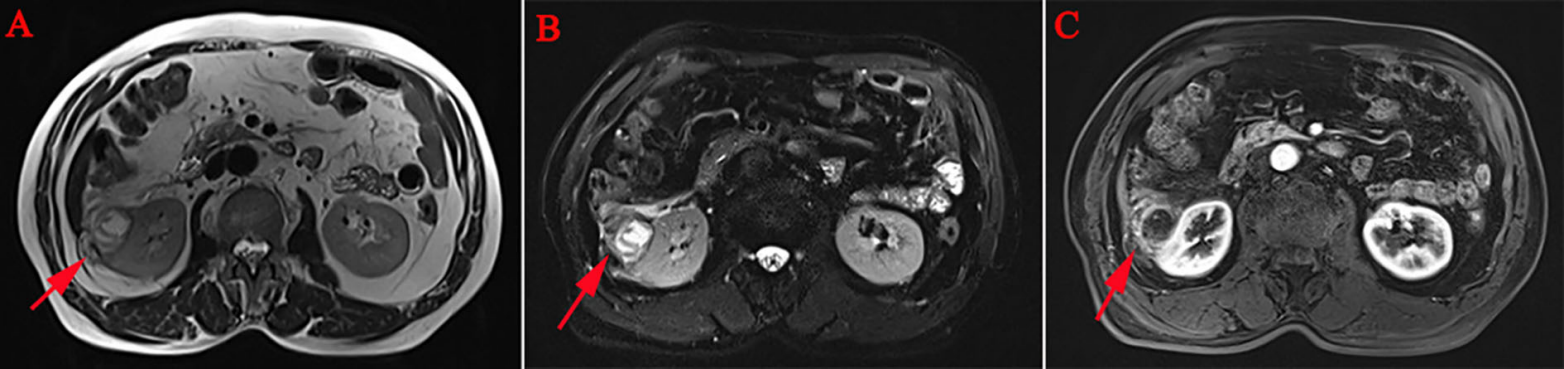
Abdominal MRI: This image showed flocculent, nodular, and cord-like mixed-signal lesions in the right perirenal space. The largest nodule measured approximately 3.0 cm × 2.4 cm with ill-defined borders, causing slight compression of the right kidney, and the solid components of the lesions exhibited enhancement. In addition, the right lateral conical fascia and prerenal fascia were thickened, with clearly enhancing nodular shadows observed within them [(A–C), red arrow].
